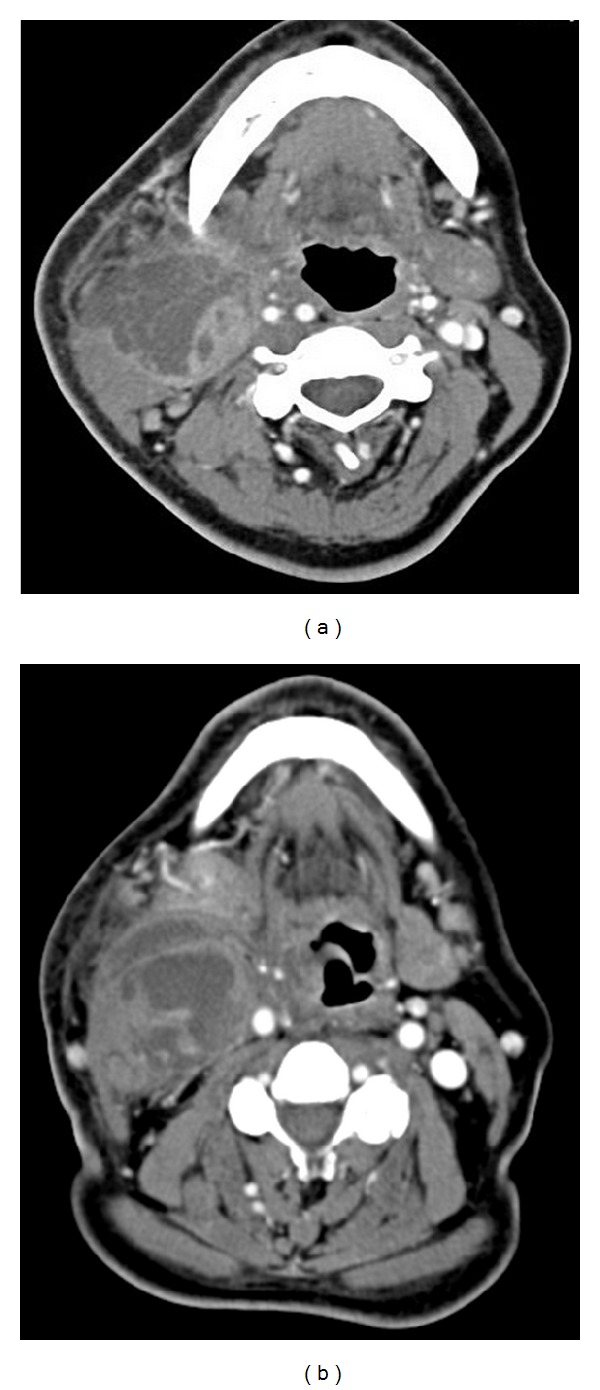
Neck mass imaging. (a) Computed tomography appearance in Case 1 of the multiloculated abscess involving the right sternocleidomastoid muscle. (b) Computed tomography appearance in Case 2 of the multiloculated abscess involving the right parapharyngeal space. Both patients required surgical drainage for pathogen identification and treatment.
